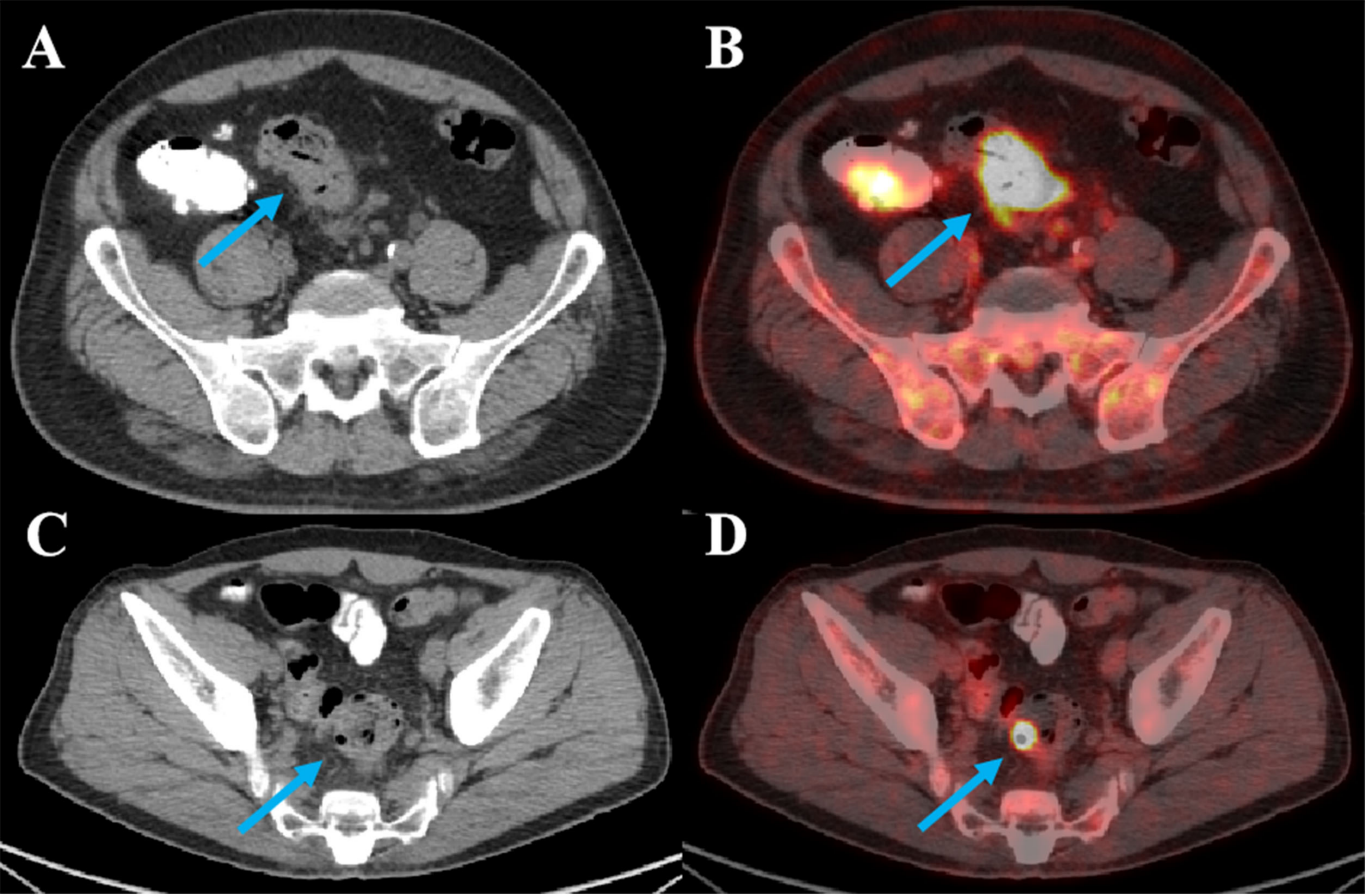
Representative ^18^F-FDG PET/CT images of colorectal carcinoma and adenoma. (A, B) A 51-year-old male patient with carcinoma of sigmoid colon. The tumor showed strong FDG uptake with SUVmax of 16.6, SUVmean of 8.3, MTV of 12.7, and TLG of 105.4. (C, D) A 61-year-old male patient with tubulovillous adenoma of sigmoid colon. The tumor also showed strong FDG uptake with SUVmax of 25.6, SUVmean of 15.3, MTV of 0.3, and TLG of 4.0.
